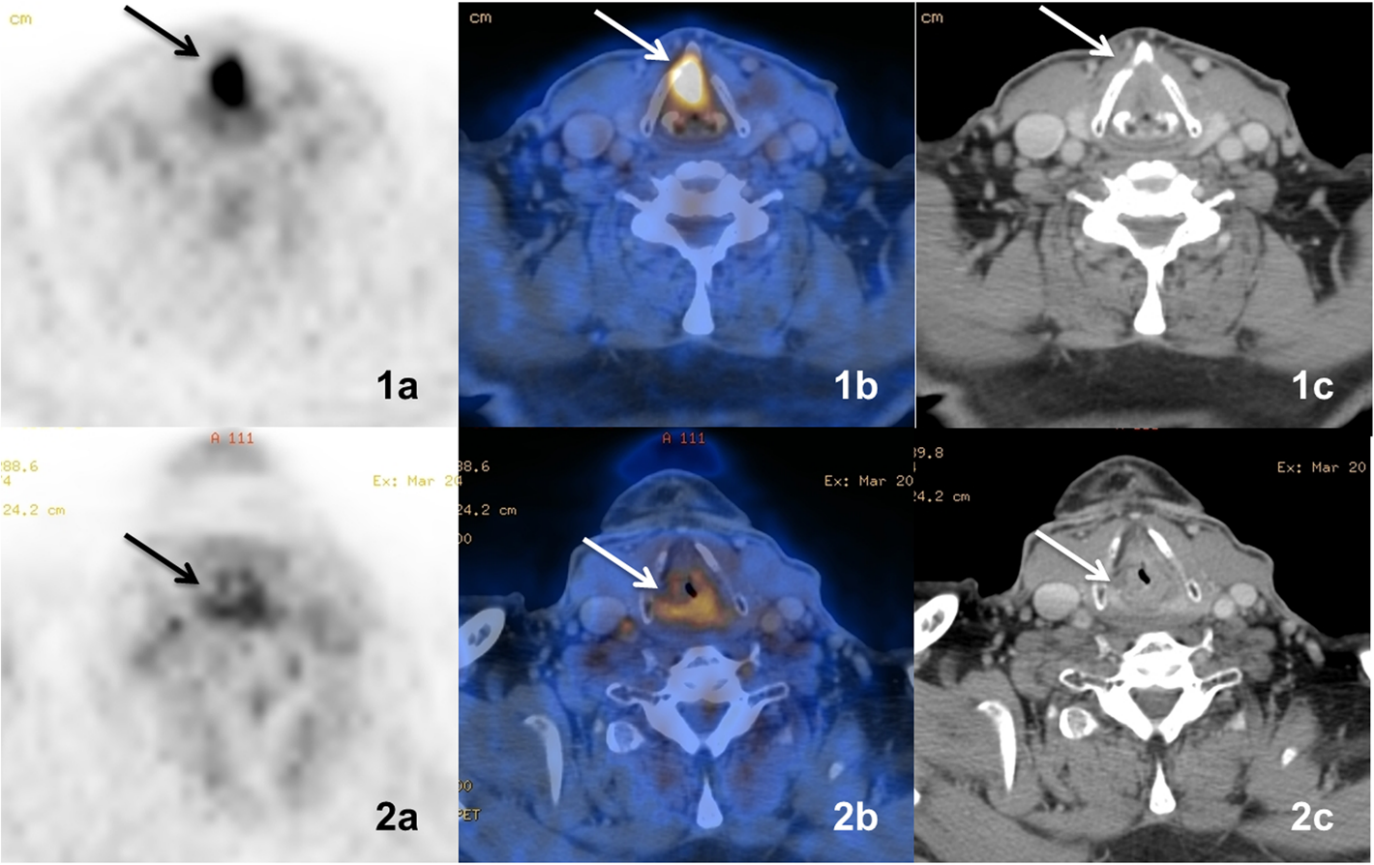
71 yo male with squamous cell carcinoma of the larynx. PET/CT. 1a–c axial PET, PET/CT, CT with contrast. Pre-therapeutic scan showing the tumor (*arrow*). 2a–c axial PET, PET/CT, CT with contrast. Post-therapeutic scan showing the residual reactive changes without tumor (*arrow*)
